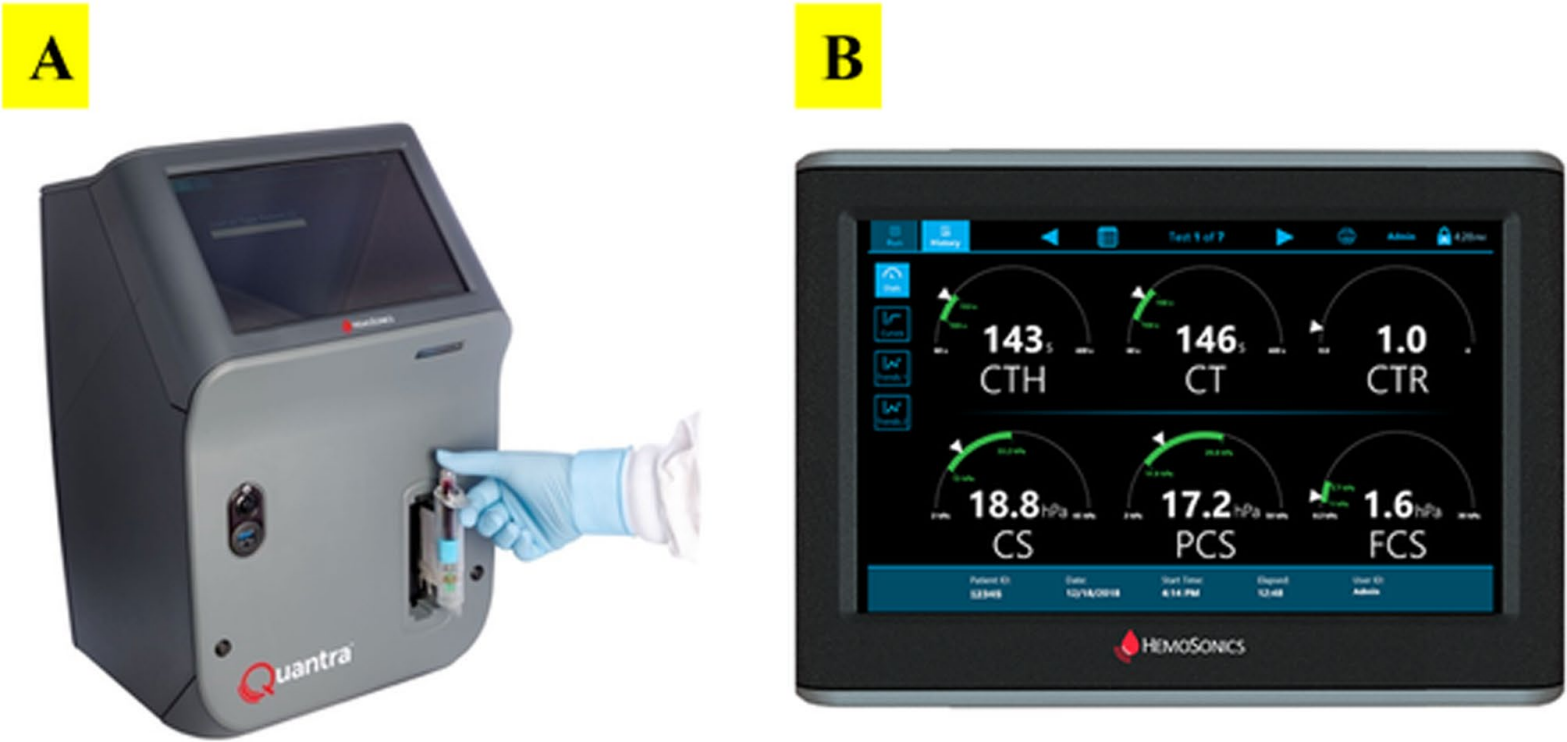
(A) Quantra device with insertion of an inverted blood sample (B) The screen of the Quantra device displays 6 parameters: Clot Time with Heparinase (CTH), Clot Time (CT), Clot Time Ratio (CTR), Clot Stiffness (CS), Platelet Contribution to clot Stiffness (PCS), Fibrinogen Contribution to clot Stiffness (FCS)
